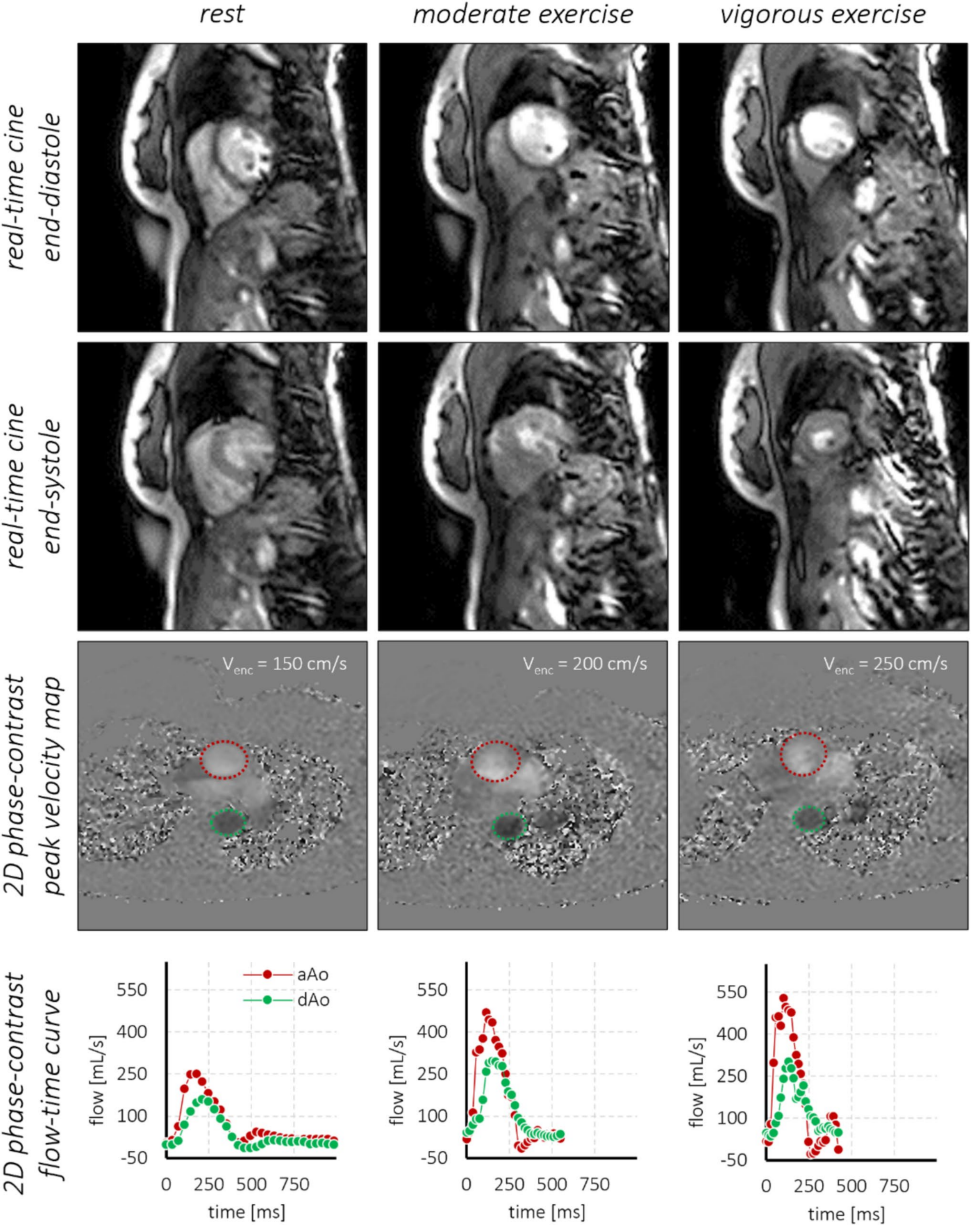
Exercise MRI stress testing in a female volunteer (age, 30 years). Real-time cine MRI at 3 Tesla was used to acquire a stack of short-axis cine MRI series at rest (heart rate, 58 beats/min), during steady-state moderate-intensity bicycling exercise (workload, 60 W; heart rate, 105 beats/min), and during steady-state vigorous-intensity bicycling exercise (workload, 115 W; heart rate, 136 beats/min). Cine MRI series were used for volumetric quantifications, and 2D phase-contrast MRI was used to quantify blood flow through the aorta. Series are available as videos in the Online Supplementary Information
